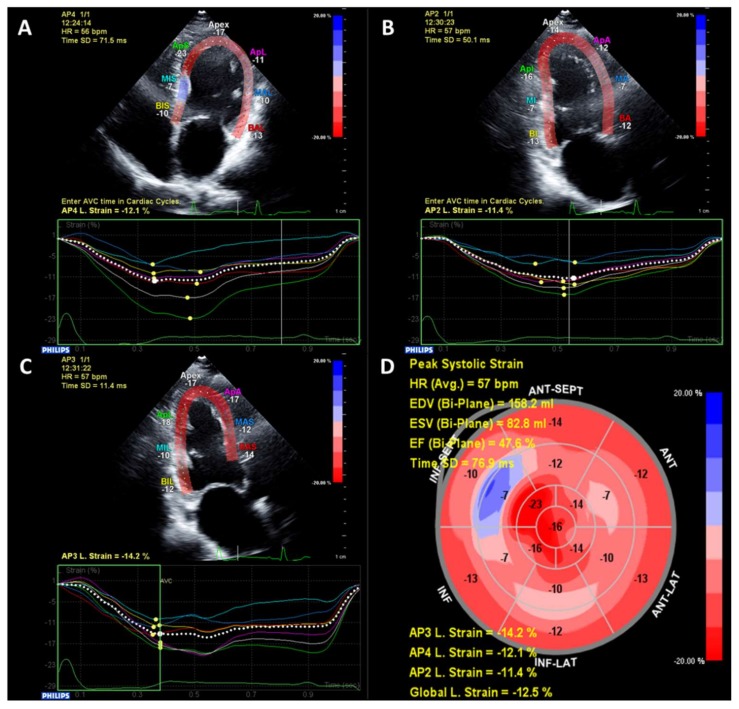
An example of echocardiographic speckle-tracking strain analysis. Images are taken in the apical 4-chamber (A), 2-chamber (B) and 3-chamber (C). A bullseye plot combining all three views is generated with reduced global longitudinal strain of −12.5% and reduced LVEF (47.6%) (D).
